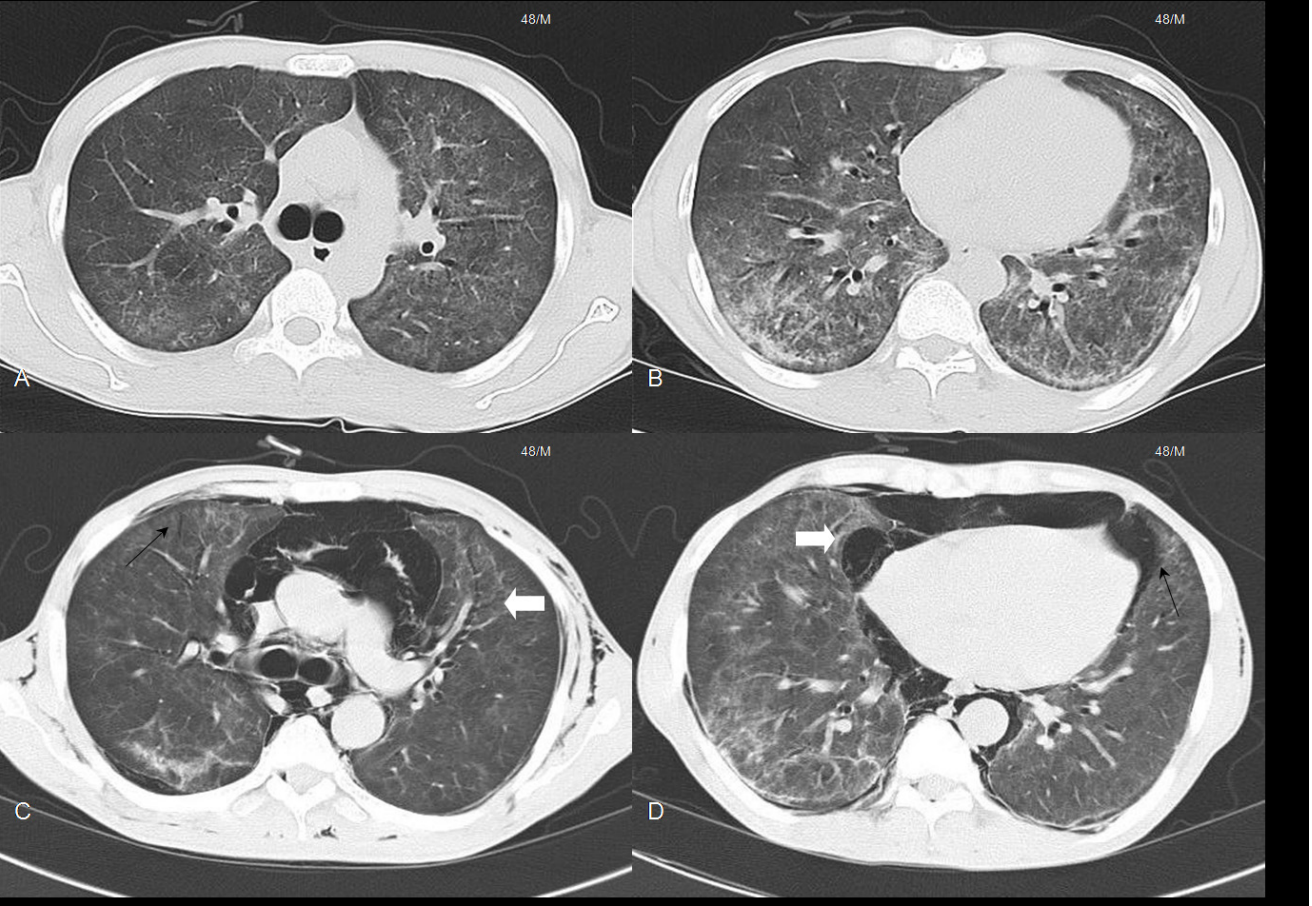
Radiological findings of an 48-year-old man with *Pneumocystis jirovecii *pneumonia on admission and at follow up. A. Computed tomography on admission reveals diffuse bilateral infiltrates. B. Diffuse ground glass opacities are noted on both lung fields on admission. C. High resolution computed tomography reveals newly developed bronchiectatic changes (white arrow) and parenchymal tears (black arrow) at follow up. D. Pneumomediastinum (black arrow) and cystic changes (white arrow) are seen at follow up.
